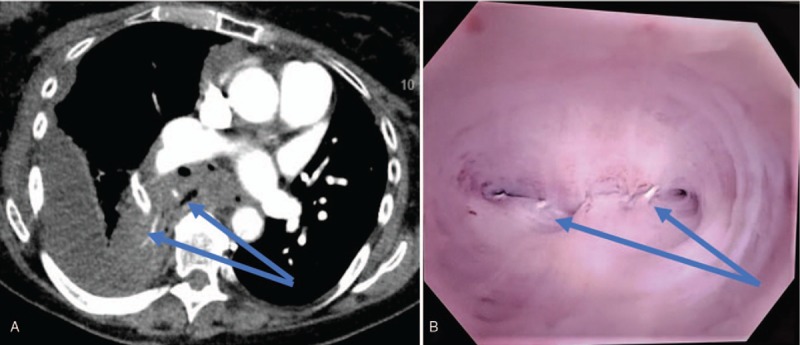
A. CT scan showing consolidated SS of RLL and proximal staple line; B. Bronchoscopic view showing erroneous transection of SS bronchus with obvious staples.
